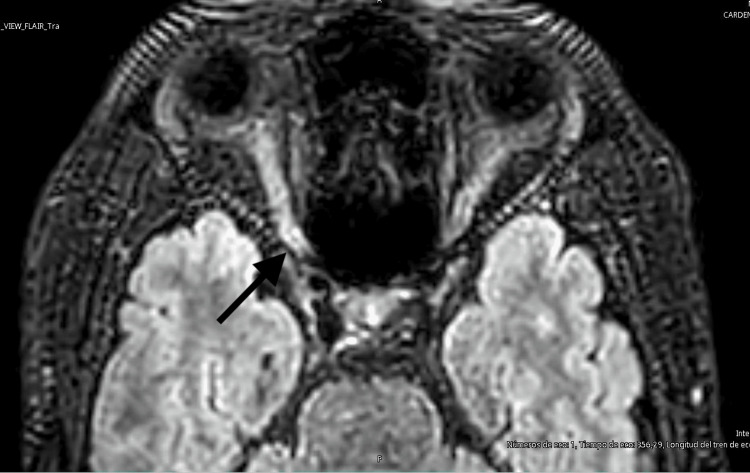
Brain MRI, non-contrast, T2-weighted FLAIR sequence, demonstrating hyperintensity in the intraorbital portion of the right optic nerve MRI: magnetic resonance imaging; FLAIR: fluid-attenuated inversion recovery
